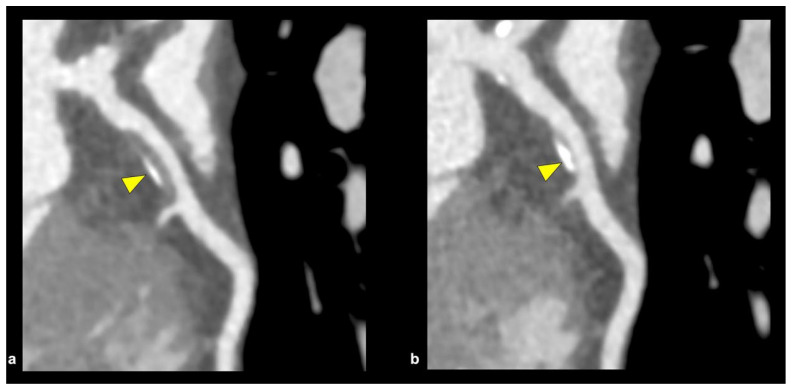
Morphological physical change in coronary artery plaque seen on CCTA, showing a partially calcified plaque in the proximal LAD artery. (a) The arrowhead depicts plaque size prior to the initiation of optimal medical therapy. (b) The arrowhead depicts coronary plaque has downsized and the calcified portion has increased after 3 years on optimal medical therapy. CCTA = coronary computed tomography angiography; LAD = left anterior descending. Source: Yoshida, K.; Tanabe, Y.; Hosokawa, T.; Morikawa, T.; Fukuyama, N.; Kobayashi, Y.; Kouchi, T.; Kawaguchi, N.; Matsuda, M.; Kido, T.; et al. Coronary computed tomography angiography for clinical practice. *Jpn. J. Radiol.*
